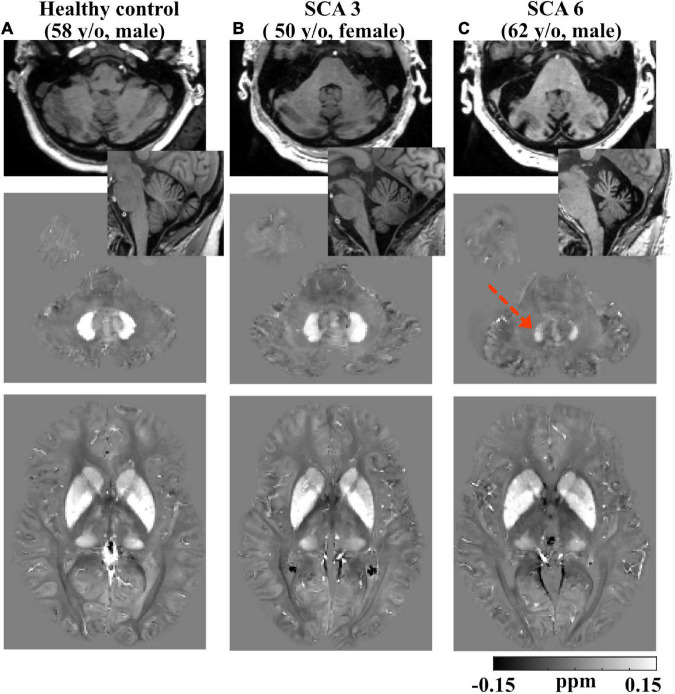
Example T1-weighted and QSM images. Row 1: axial and sagittal T1-weighted images showing smaller total cerebellar volume in a (62 y/o, M) SCA6 subject (C) vs. a (50 y/o, F) SCA3 subject (B) and a (58 y/o, M) healthy control subject (A); slight atrophy in cerebellum was also observed in SCA3. Row 2: axial QSM images showing the dentate. Dotted arrow denotes lower QSM and atrophied dentate in the SCA6 subject. Row 3: axial QSM showing the basal ganglia. Gray scales in QSM images are in the range of (–0.15, 0.15) ppm. All the images are rigidly transformed to MNI space for better comparison of the same anatomical structures.
